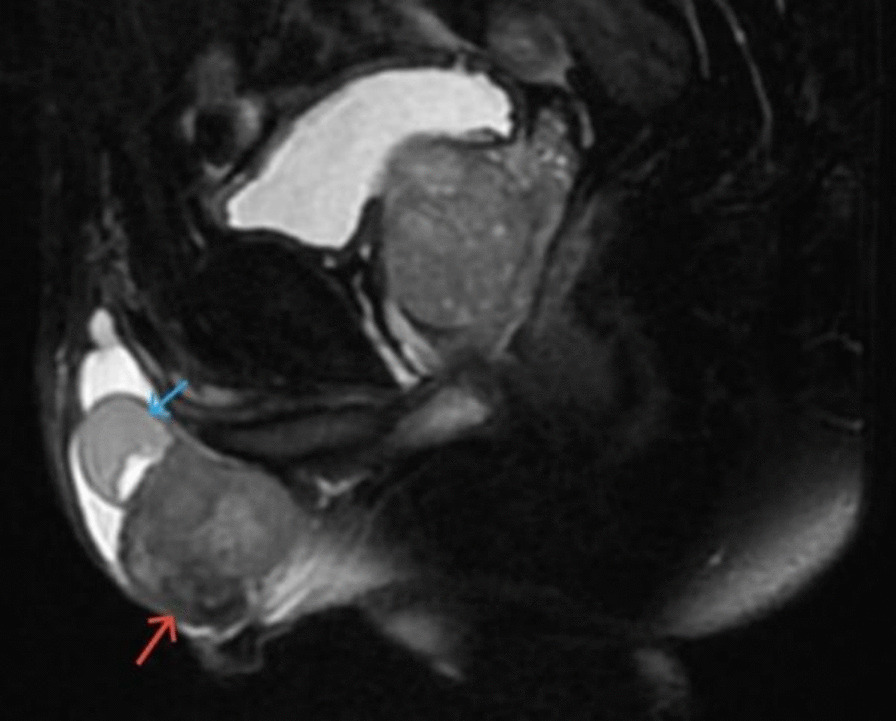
Magnetic resonance imaging showing that the mass (red arrow) around the right testicle (blue arrow) was about 3.5 cm × 3.5 cm × 4.0 cm in size and oval in shape
